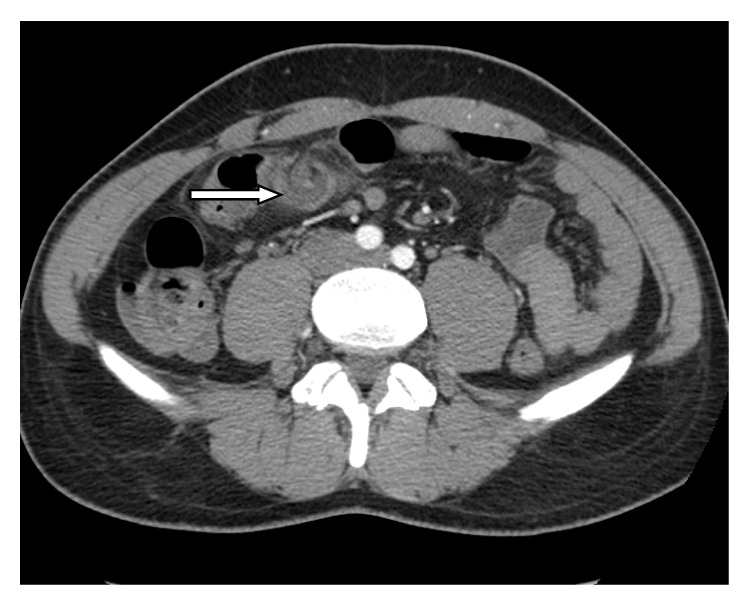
Axial contrast-enhanced CT scans obtained at the twisted point of omentum.
